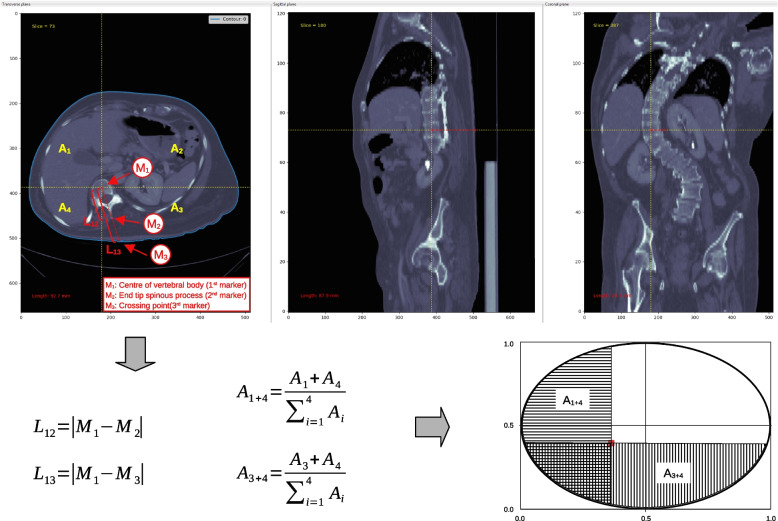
Annotated scheme of the data analysis at the example of a strong spinal deformation seen in computed tomography. At the transverse view, arrows denote the marker positions at the centre of the vertebral body, at the end tip of the spinous process and at the crossing point between the straight through the previous, two markers and the body contour. $$A_1$$...$$A_4$$ denote the areas of the four quadrants enclosed by the body contour (blue line), which were used to find the corresponding position inside the ellipse
